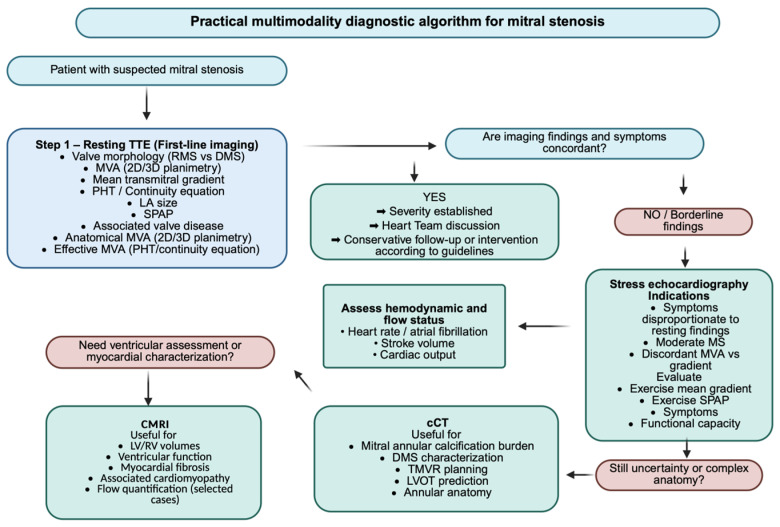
Practical multimodality diagnostic algorithm integrating echocardiography, stress echocardiography, cardiac CT, and cardiac magnetic resonance in the evaluation of mitral stenosis. Abbreviations: cCT: cardiac computed tomography; CMR: cardiac magnetic resonance; DMS: degenerative mitral stenosis; LA: left atrium; LV: left ventricle; LVOT: left ventricular outflow tract; MAC: mitral annular calcification; MR: mitral regurgitation; MS: mitral stenosis; MVA: mitral valve area; PH: pulmonary hypertension; PHT: pressure half-time; PMC: percutaneous mitral commissurotomy; RMS: rheumatic mitral stenosis; SPAP: systolic pulmonary artery pressure; TMVR: transcatheter mitral valve replacement; TTE: transthoracic echocardiography.
